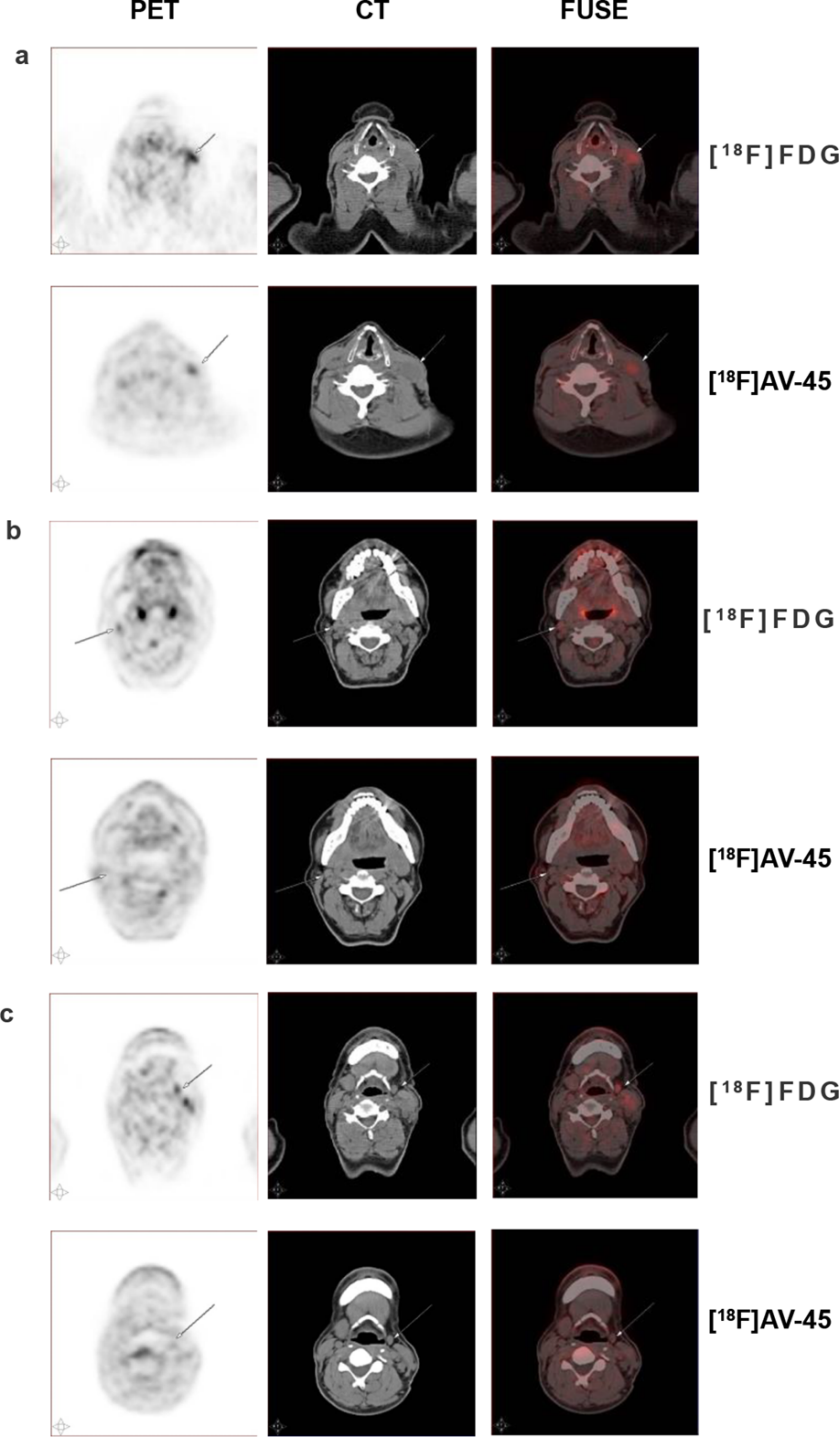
[^18^F]FDG and [^18^F]AV-45
